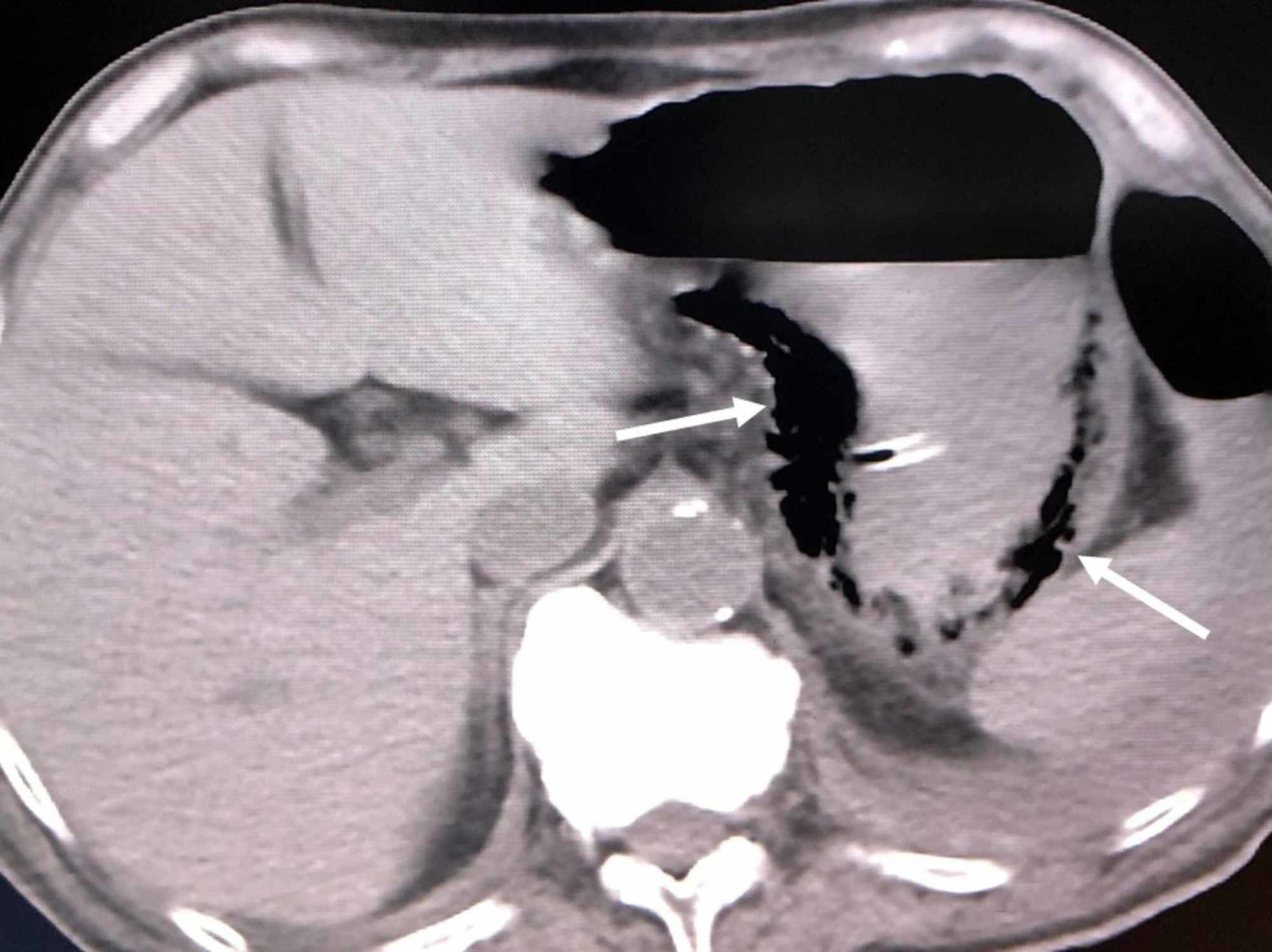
Computed tomography (CT) on the 28th hospital day (arrows) CT revealed left pyothorax and emphysematous gastritis.
